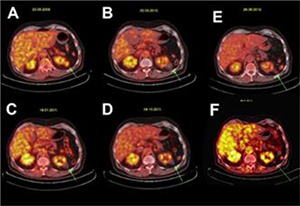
Consecutive ^18^F-FDG PET-CT imaging of a renal cancer before and after cryoablation: Green arrows indicate the tumor or the ablation site in the left kidney of patient number 4. Before surgery, (A) there was metabolic activity noticed in the tumor. The low-dose CT scans showed consecutive decrease in the tumor volume, and no metabolic activity was measured in the tumor using ^18^F-FDG PET at 5 months (B), 15 months (C), and 24 months (D) follow-up after LCA. However, at 34 months, newly developed metabolic activity was noticed in the perirenal fat that covered the ablated tumor (green arrow panel E). Spontaneous disappearance of metabolic activity in the perirenal fat at 63 months (panel F).
